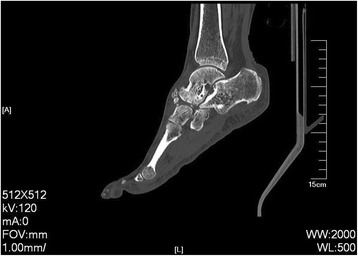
CT scan showing degenerative changes in the subtalar joint
